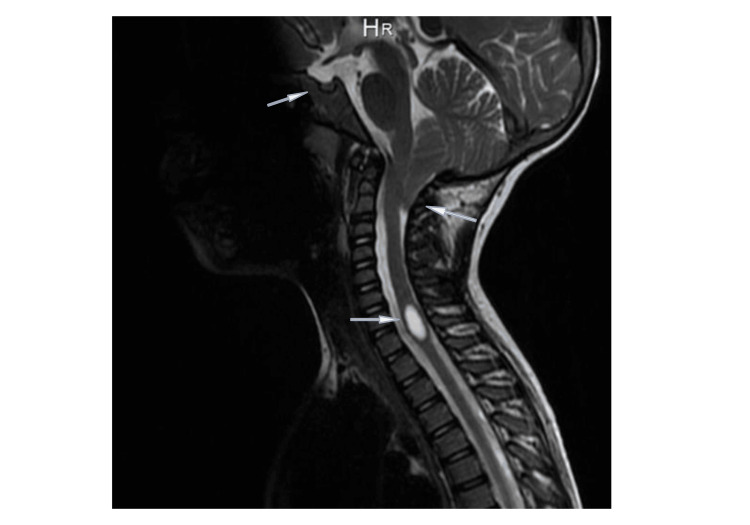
MRI of the first patient before the initiation of GH therapy, which presents a slight prolapse of the cerebellar tonsils, syringomyelia in the cervical spine, and a pituitary with slightly smaller measurements than normal. MRI: Magnetic Resonance Imaging, GH: Growth Hormone
